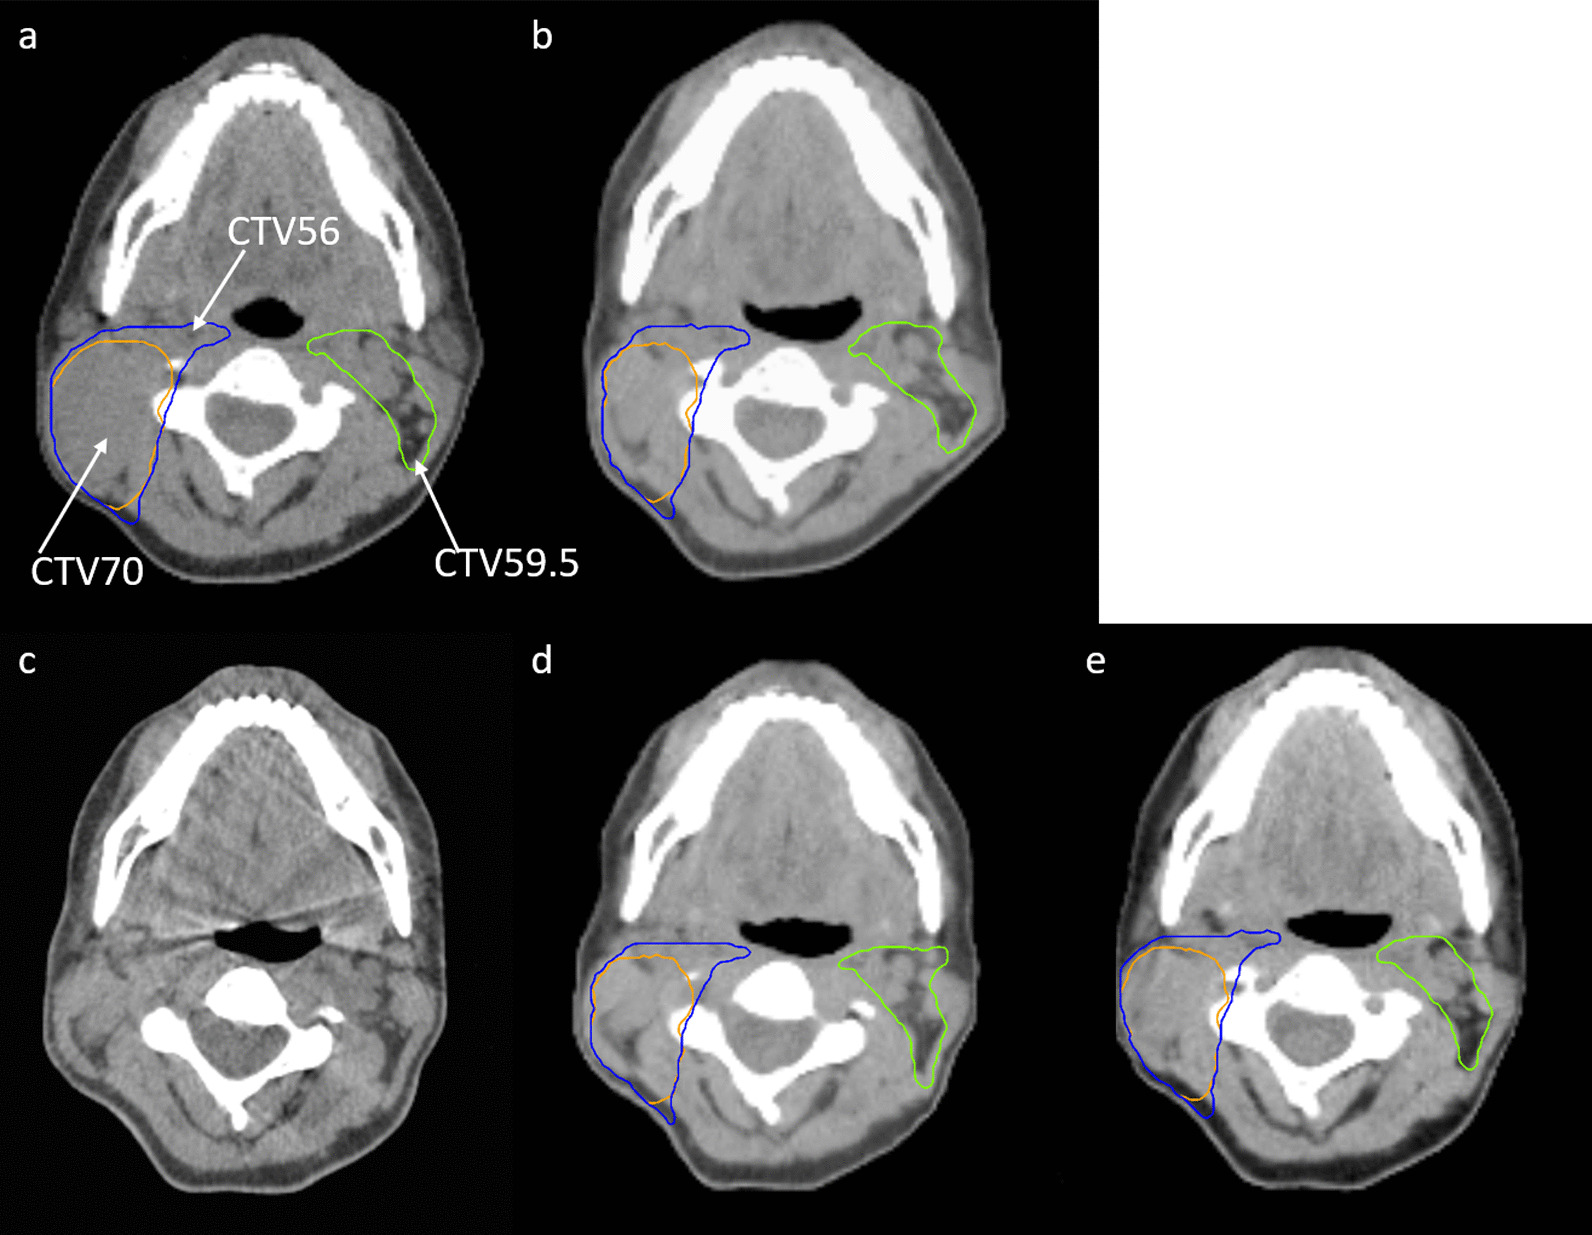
An example case with different CT images. a pCT; b rCT; c CBCT; d rCT_def_ with gold standard contours; e sCT with DIR-propagated contours
